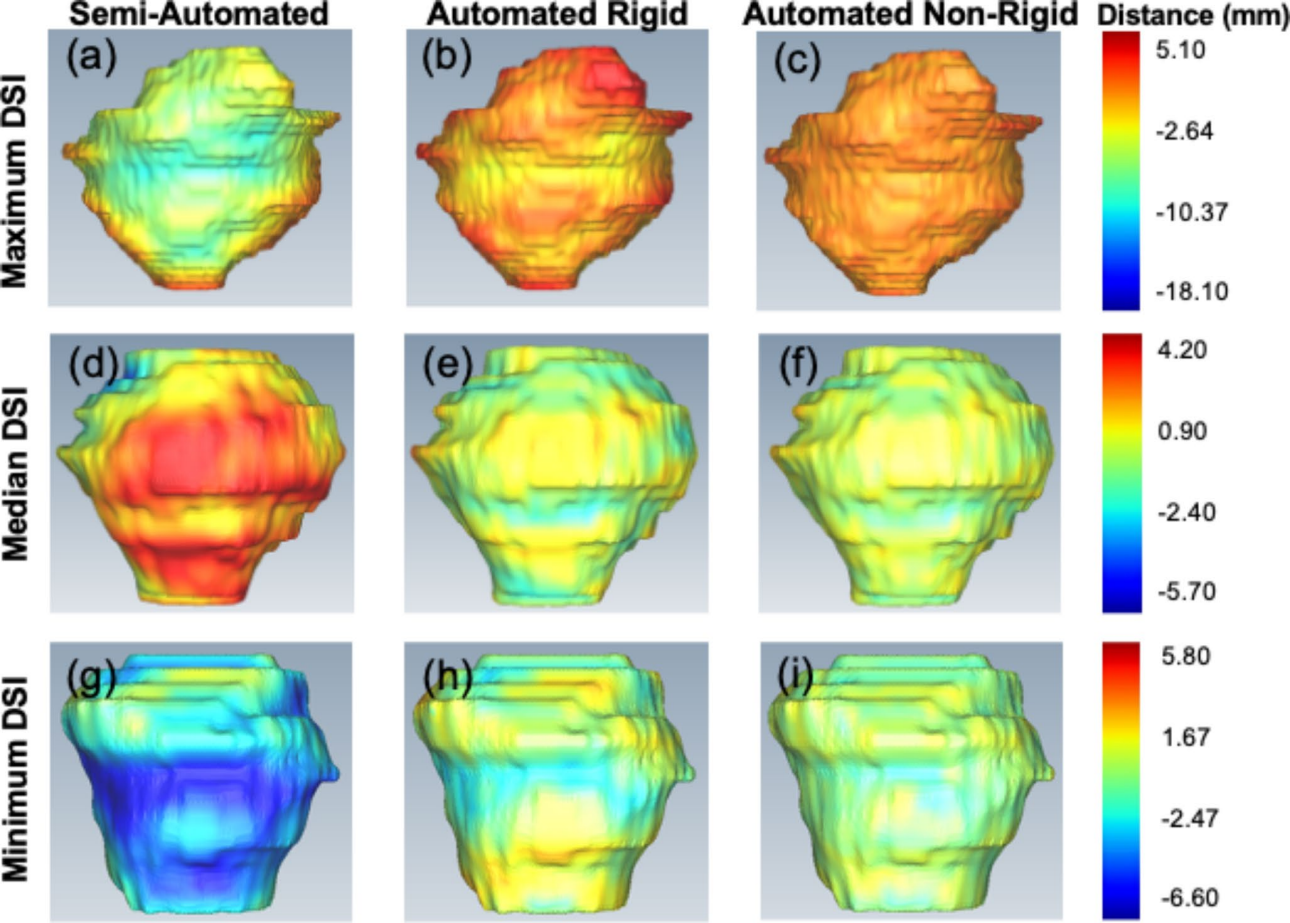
Coronal view prostate surface distance between the manual MR contours and the contours generated through the registration of the CT and MR images. Columns 1 (a, d, g), 2 (b, e, h) and 3 (c, f, i) display the semi-automated rigid-, automated rigid- and automated non-rigid-registered results, respectively. Rows 1 (a-c), 2 (d-f) and 3 (g-i) display the case which achieved the maximum-, median- and minimum DSI result using the automated non-rigid registration method, respectively. The signed distance scalar bar (rainbow colour map on right side of each row) has units in mm. MR, magnetic resonance; CT, computed tomography; DSI, dice similarity index
